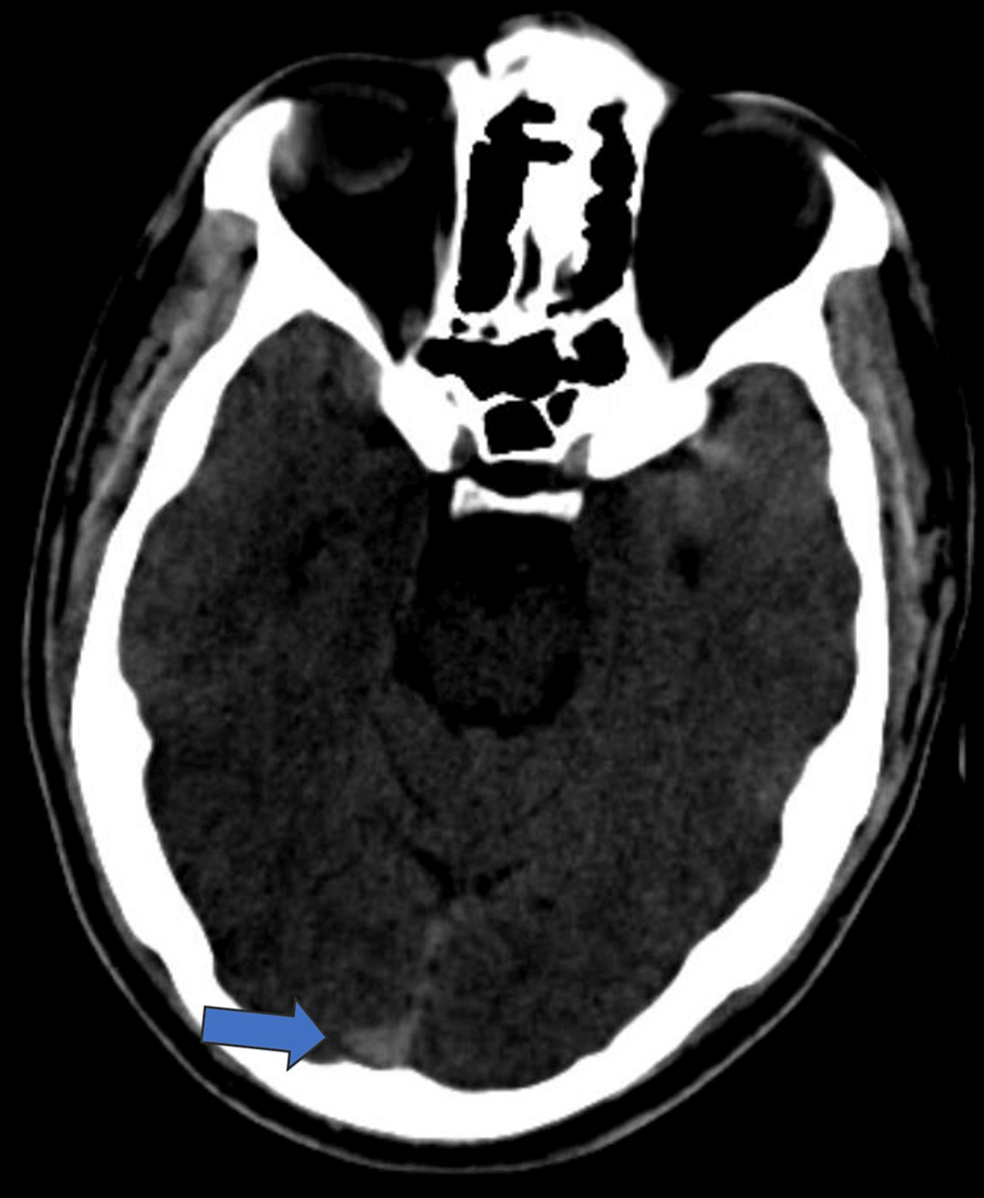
CT brain in axial view showing dense superior sagittal sinus (blue arrow) CT: computed tomography
